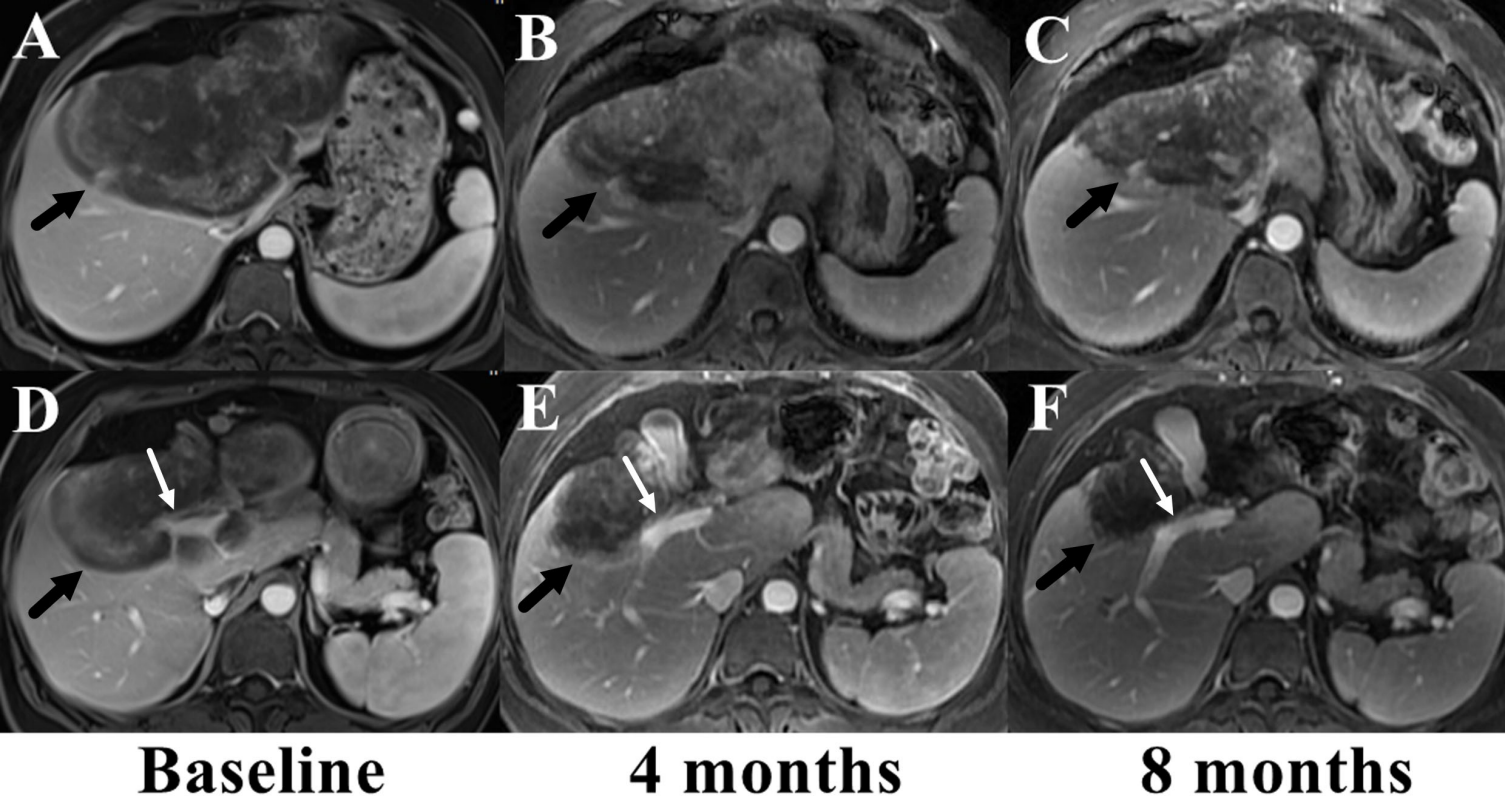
Comparison of the largest lesion on MRI before (A, D) and after the combined therapy of sirolimus and IFN-a 2b (B, C, E, F). The tumor (marked with black arrows) gradually regressed and the involved right branch of portal vein (marked with white arrows) got released from the tumor.
